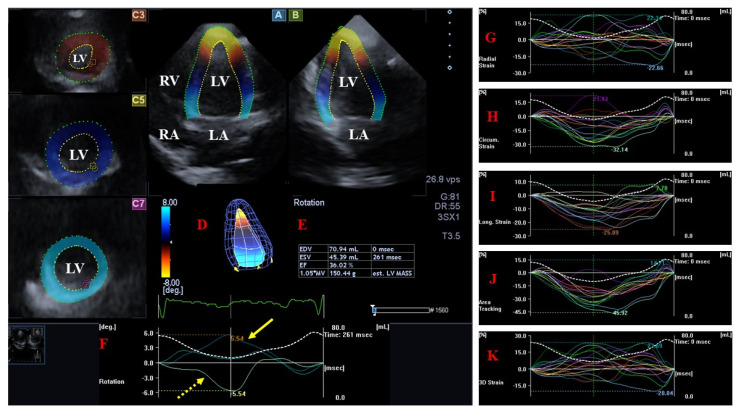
Evaluation of the left ventricle by three-dimensional (3D) speckle−tracking echocardiography. Following the acquisition of a 3D echocardiographic dataset and by using a dedicated vendor-provided software, several views are produced automatically: longitudinal apical four-chamber (A) and two-chamber (B) views and short-axis views at the apical (C3), midventricular (C5), and basal (C7) LV regions. The 3D LV model (D), calculated LV volumes and ejection fraction (E), and apical (yellow arrow) and basal (yellow dashed arrow) LV rotations (F), together with time–LV global (white curve) and segmental (colored curves) radial (G), circumferential (H), longitudinal (I), area (J), and 3D (K) strain curves with a time–LV volume change curve (dashed white curve), are demonstrated. The image presents a patient with reduced LV function (decreased LV ejection function) and normally directed LV rotational mechanics with reduced counterclockwise apical (yellow arrow) and preserved clockwise basal (dashed yellow arrow) LV rotations. Abbreviations: LV = left ventricle, LA = left atrium, RV = right ventricle, RA = right atrium, EDV = LV end-diastolic volume, ESV = LV end-systolic volume, EF = LV ejection fraction, MASS = LV muscle mass.
